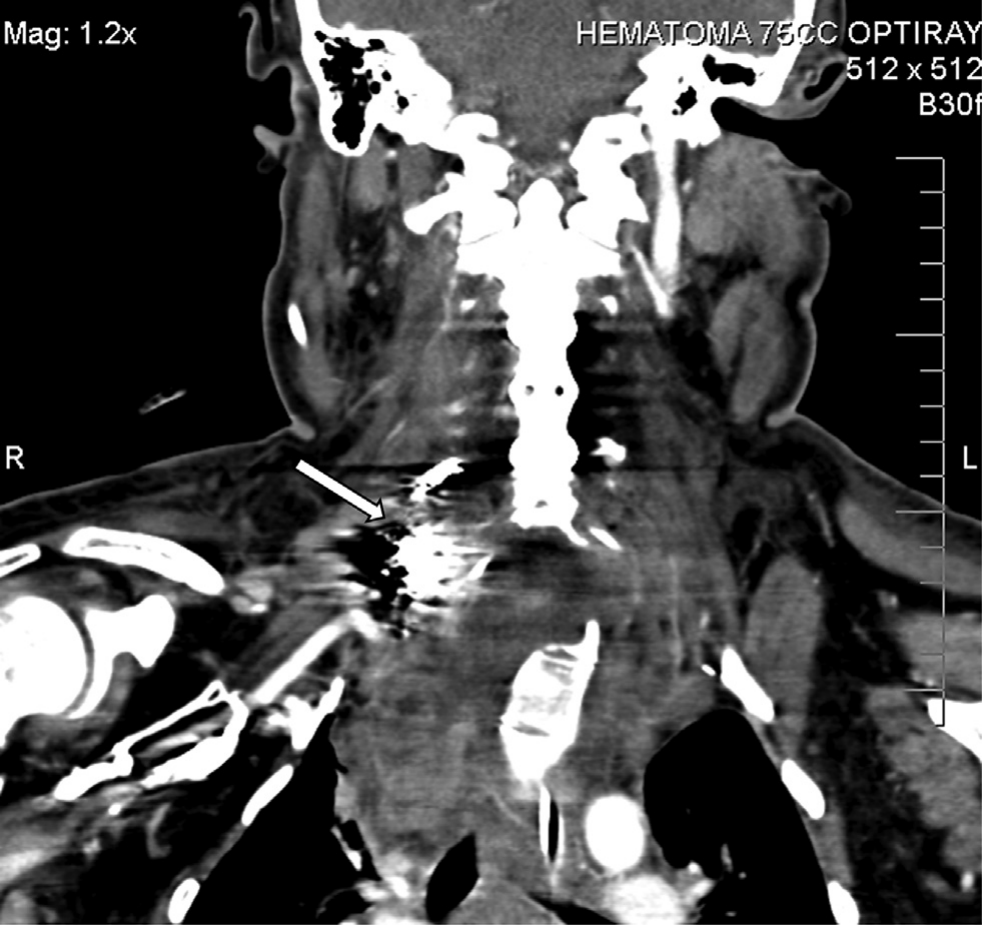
CT Neck status post coiling and hematoma evacuation, revealing embolic coil material in the proximal right vertebral artery. Hematoma decreased in size from Fig. 1 with decrease in degree of tracheal deviation.
